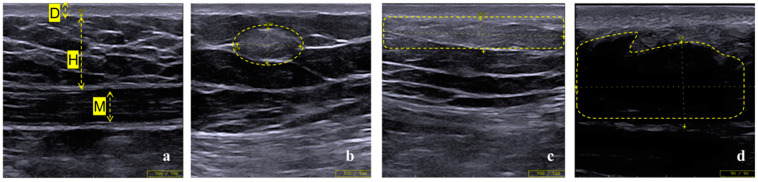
Imaging features of normal tissue and LH under ultrasound. (a) D, dermis; H, hypodermis (mainly adipose tissue); M, muscular layer. Each layer is clearly demarcated. (b) Nodular hyperechoic LH with obvious space occupying sensation. (c) Diffuse hyperechoic LH presented with no clear demarcation from the dermis. (d) Hypoechoic LH with irregular boundary, which is similar to adipose tissue liquefaction.
